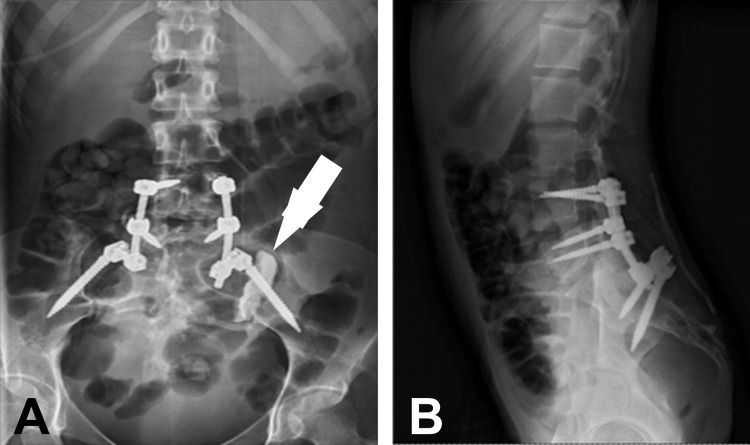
Simple radiography (A: anteroposterior, B: lateral) showing the fixation and placement of implants plus presence of bone cement (arrow) in surgical bed in the left sacroiliac joint.
